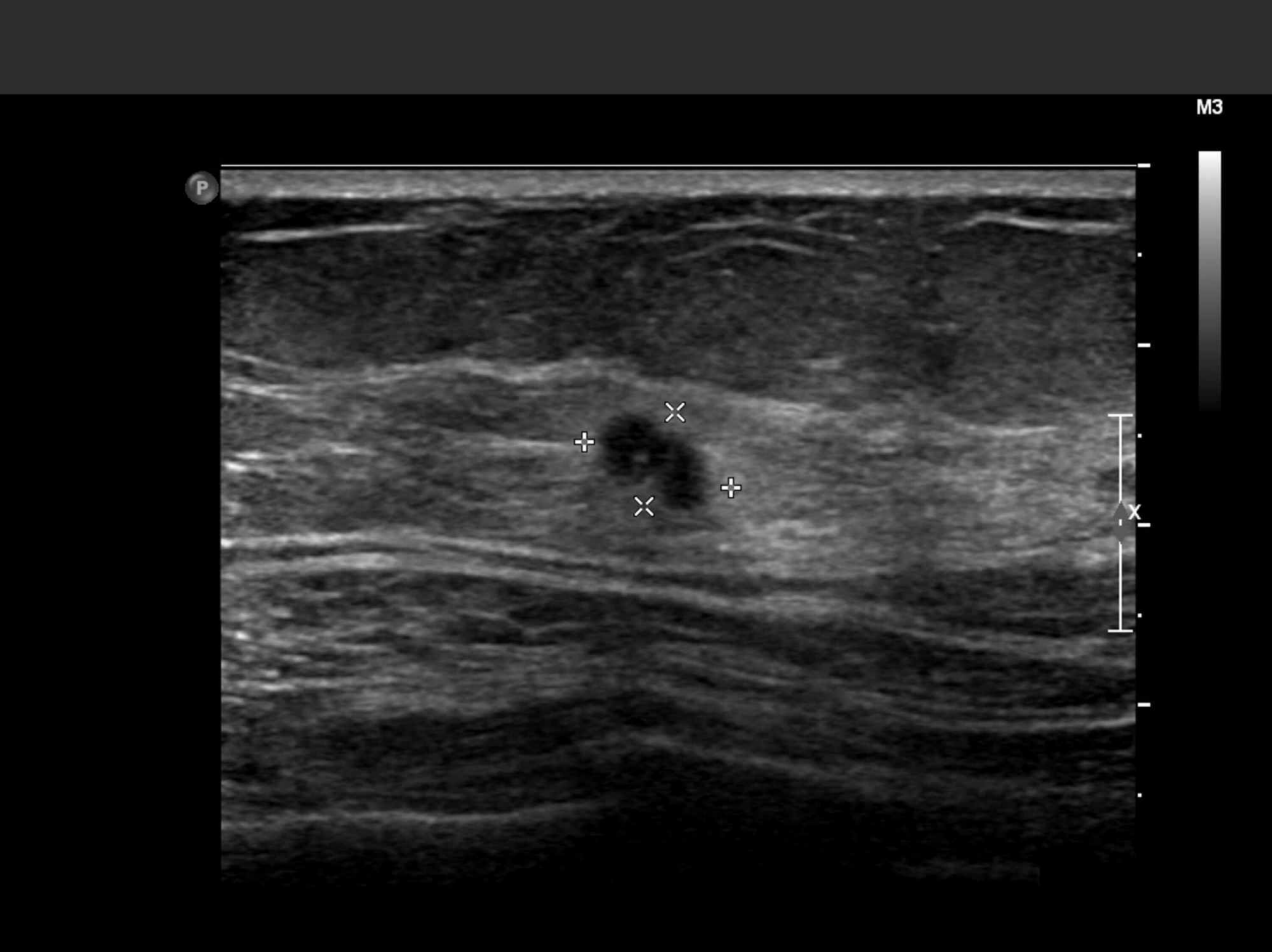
Left breast ultrasound image of the lesion.
